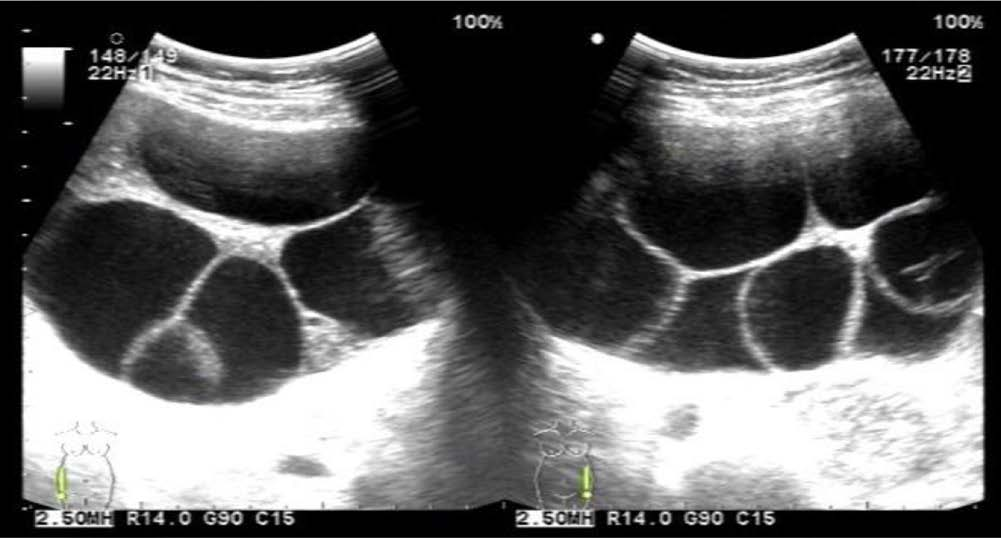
A 27-yr-old primigravida at 13 2/7 wk of gestation presented with an 8-d history of abdominal distension. Ultrasound findings indicated bilateral multiloculated ovarian cystic masses accompanied by spontaneous ovarian hyperstimulation.
